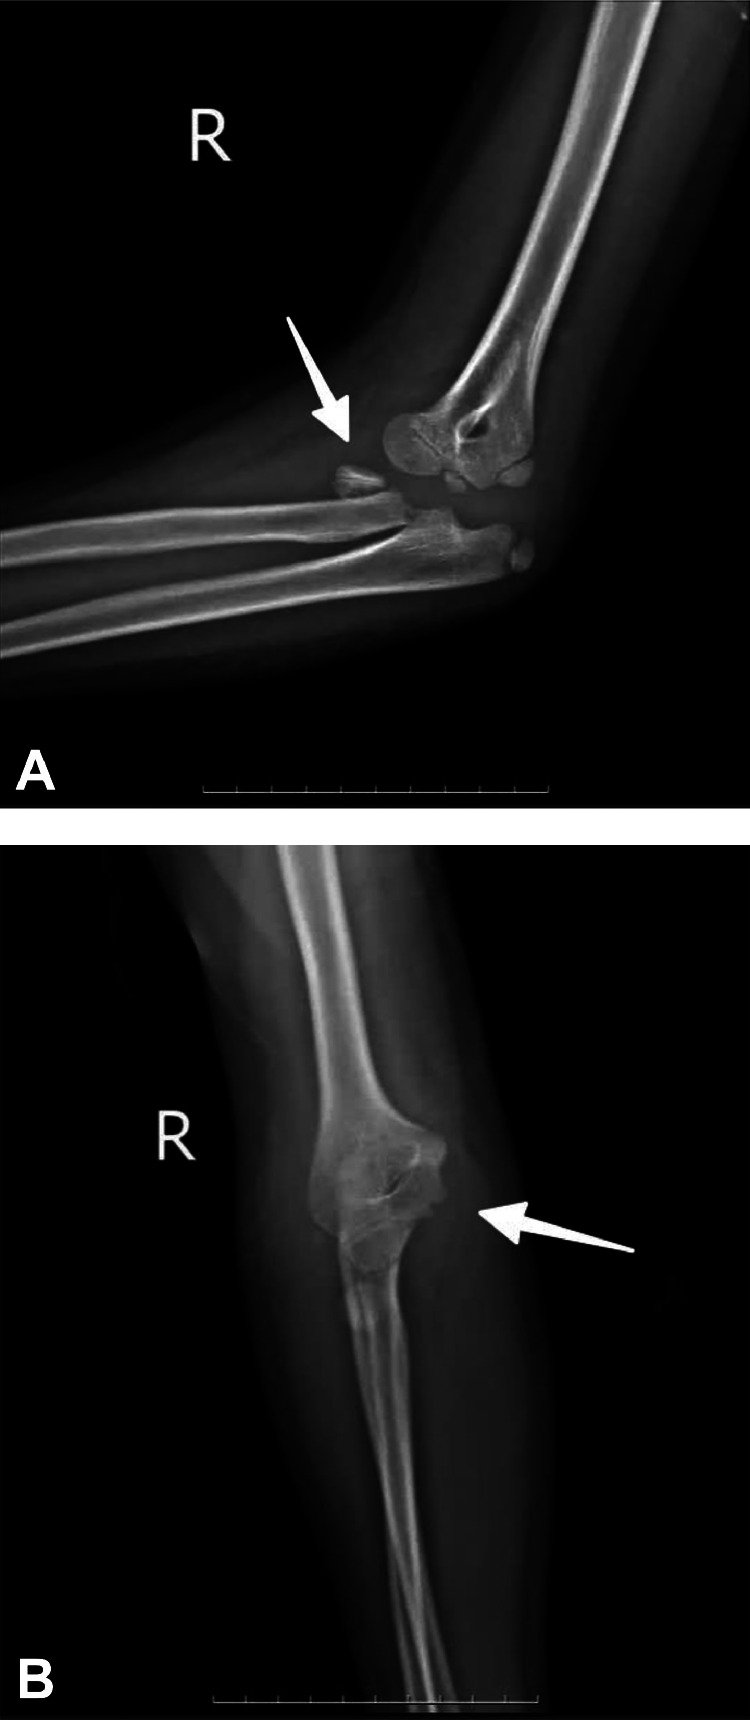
A: Initial radiographic evaluation of the right elbow Plain radiography (X-ray) in lateral view with the elbow flexed at approximately 90°, demonstrating posterior dislocation of the elbow joint with an associated radial head fracture (arrow). Note the loss of normal articulation between the distal humerus and the proximal radius and ulna, indicating posterior displacement of the forearm bones relative to the humerus. B: Initial radiographic evaluation of the right elbow Plain radiography (X-ray) in anteroposterior view with the elbow partially extended, showing medial displacement of the proximal radius and ulna. The radial head fracture is partially visualized from this view (arrow), with disruption of the normal radiocapitellar relationship.
